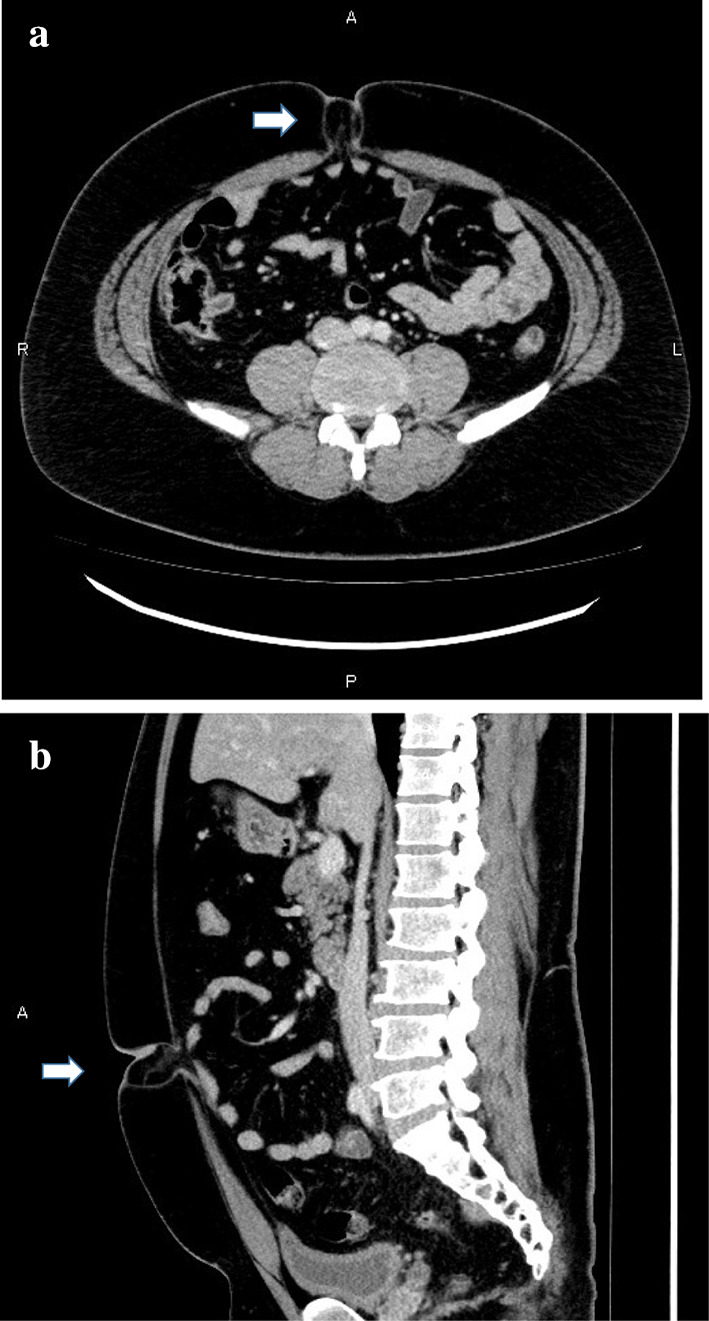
Sections of enhanced abdominal computed tomography. The greater omentum seems to be incarcerated (arrow). a Horizontal section. b Coronal section
